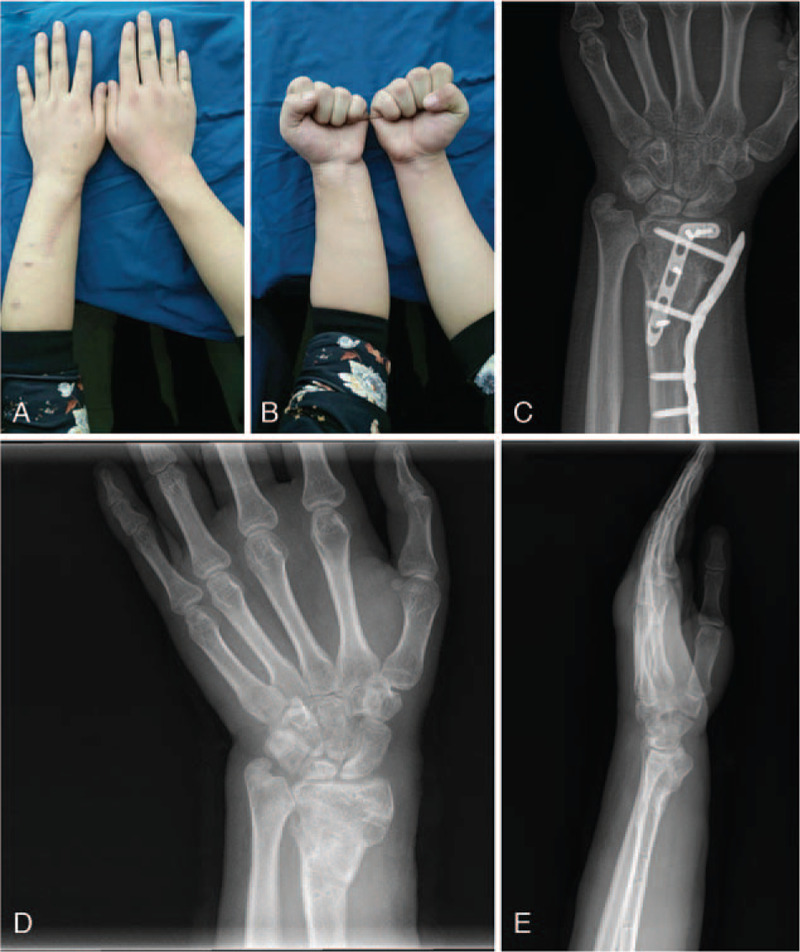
(A, B) Six months after the operation, satisfactory appearance of the wrists and (C) radiographic results of the left distal radius. (D, E) After internal fixation plates are removed, radiographic images of the wrist are gratifying.
